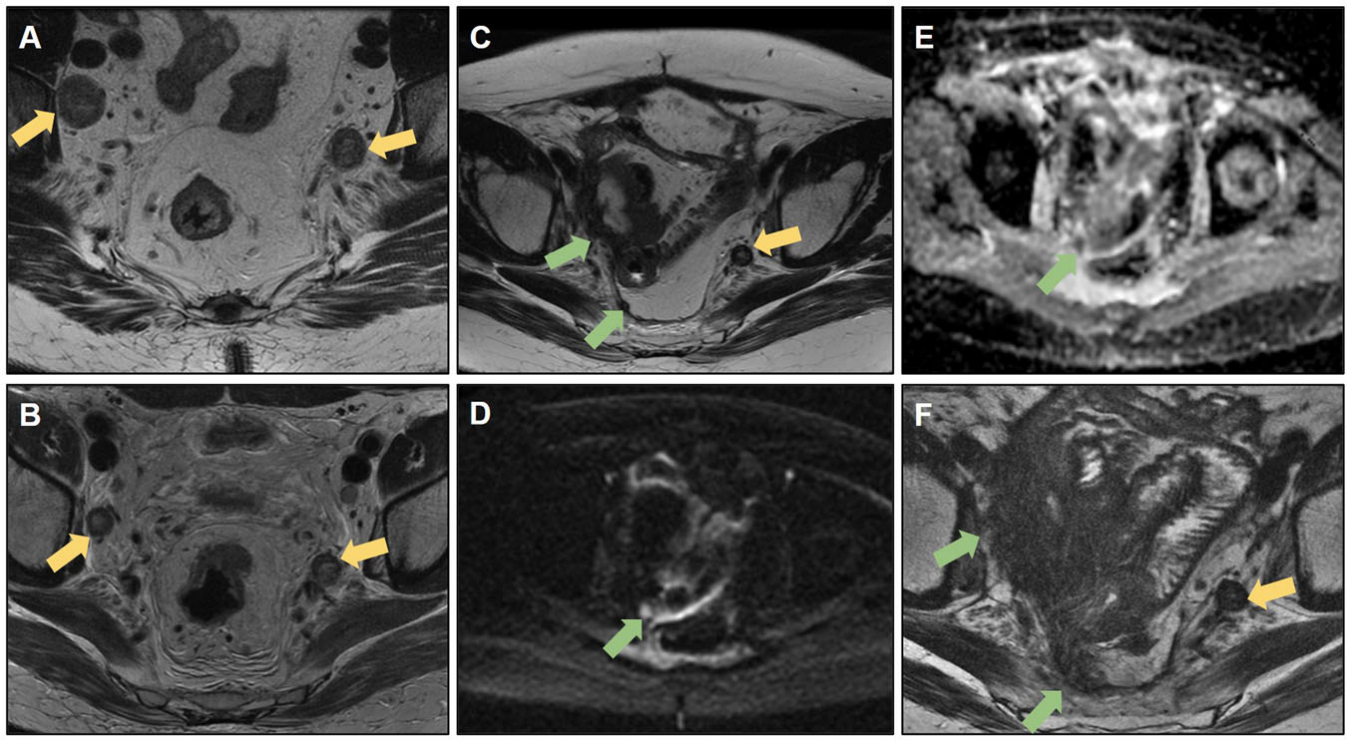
A Baseline MRI showing mrT4N1 status, with infiltration of the levator ani muscle, positive extramural vascular invasion (EMVI), involvement of the mesorectal fascia (MRF), and intersphincteric plane, with suspicious bilateral pelvic lateral lymph nodes (yellow arrow). B MRI restaging after neoadjuvant chemoradiotherapy (CRT) with a poor response (mrTRG 4), demonstrating reduction in the size of the pelvic lateral lymph nodes (yellow arrow). C Patient underwent abdominoperineal resection (APR) and bilateral pelvic lymphadenectomy; however, the persistence of a suspicious left pelvic lateral lymph node is noted (yellow arrow). Suspicious poorly defined tissue suggestive of recurrence is evident in the right pelvic sidewall (green arrow). D and E show diffusion restriction with a high signal on diffusion-weighted imaging (DWI), and a low signal on ADC of the peritoneal implant (green arrow). F Follow-up MRI revealing unresectable pelvic sidewall recurrence 1 year after surgery, with involvement of the peritoneum, bladder, seminal vesicles, and presacral space (green arrow)
